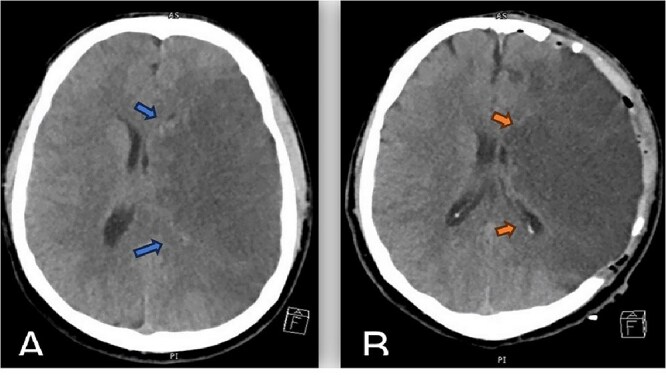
(A) CT head shows interval development of a right sided midline shift of 6.8 mm causing effacement of the left lateral ventricle. (B) Post decompressive craniectomy CT head shows interval decrease of a right sided midline shift, measuring 1.5 mm (previously 6.8 mm), with mild re-expansion of the left lateral and third ventricles.
